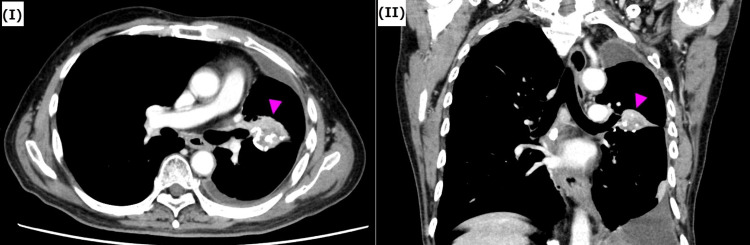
Contrast-enhanced chest CT. I: axial; II: coronal pre-treatment contrast-enhanced CT scans of the chest showing a 37×33×28 mm mass in left upper lobe lung around postoperative petz (arrowhead). Window center 30, window width 300.
